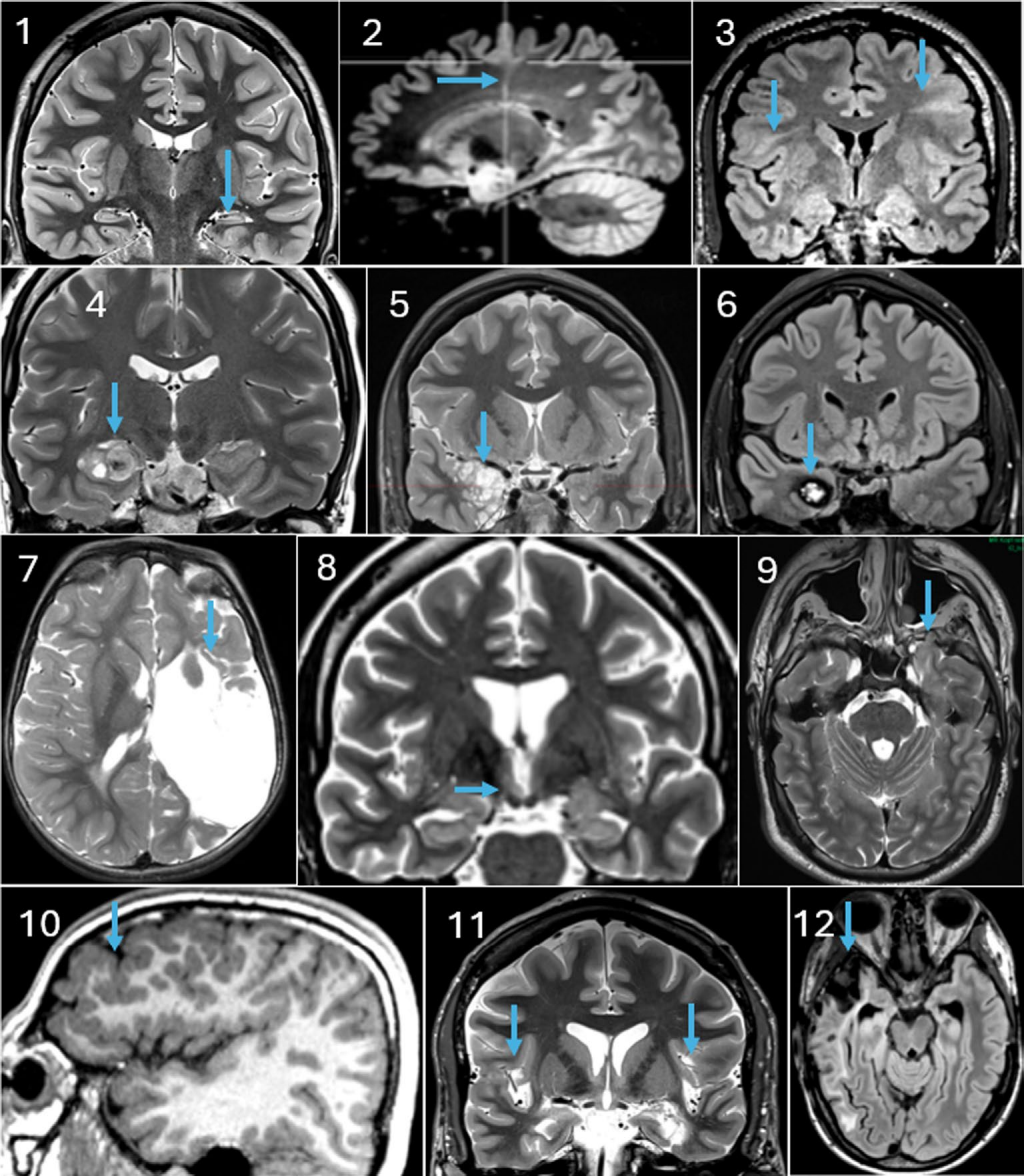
MR imaging gallery of common etiologies underlying focal epilepsy. 1. Hippocampal sclerosis, 2. FCD IIB with transmantle sign; 3. TSC with multiple tubers left and right frontal, 4. Ganglioglioma with cystic and calcified components, 5. DNET (multivacuolar), 6. Cavernoma, 7. Postischemic defect in the territory of the left MCA, 8. Hypothalamic hamartoma type II, 9. Encephalocele left temporal, 10. Frontal polymicrogyria, 11. Postencephalitic defects temporo‐insular, 12. Postcontusional defects right temporal.
